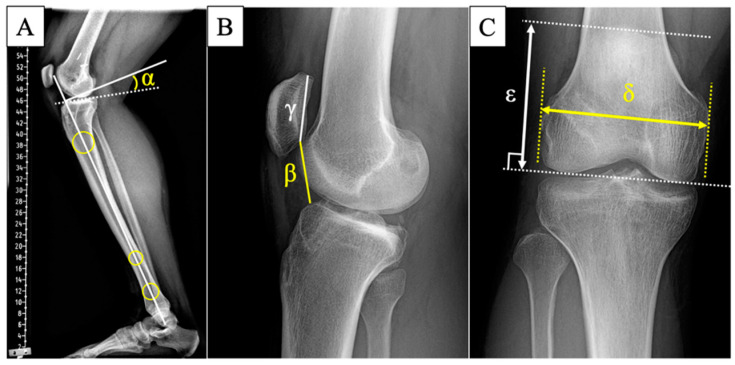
Measurement of radiographic parameters using plain radiograph. (A) posterior tibial slope = α. (B) Caton–Deschamps index = β/γ. (C) femoral patellar height index = δ/ε.
